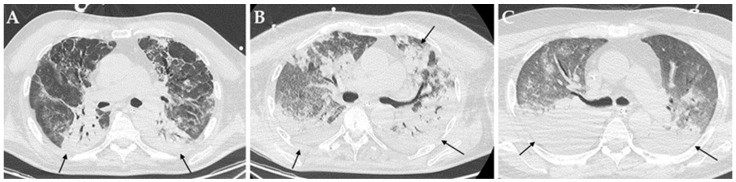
Axial HRCT images of different patients (A–C) with COVID-19 ARDS admitted to ICU showing bilateral consolidations (black arrows). In particular, all these patients developed a pulmonary superinfection by *Klebsiella pneumoniae* (A), *Pseudomonas aeruginosa* plus *Acinetobacter baumannii* (B) and *Staphylococcus aureus* plus *Candida albicans*.
